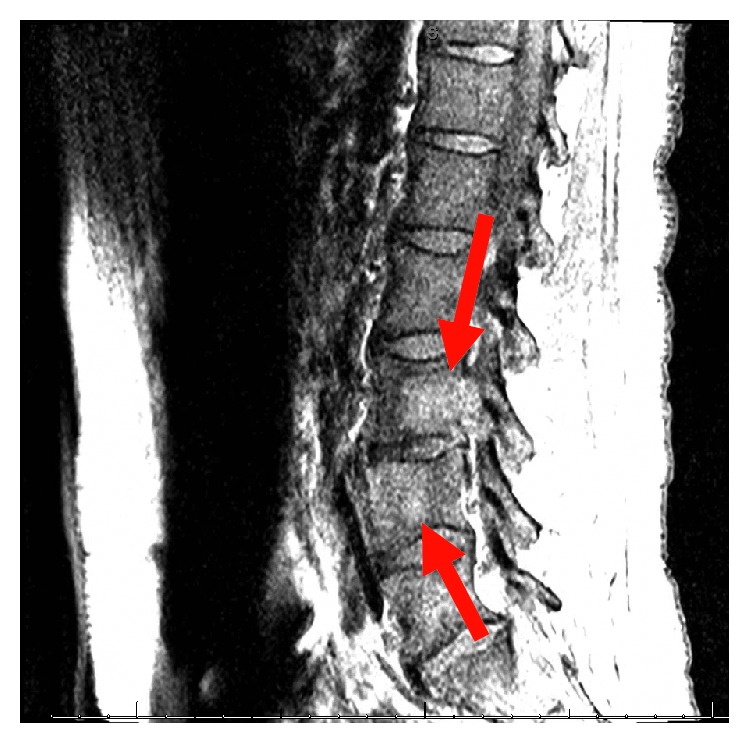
T1 sagittal segment MRI lumbar spine.
